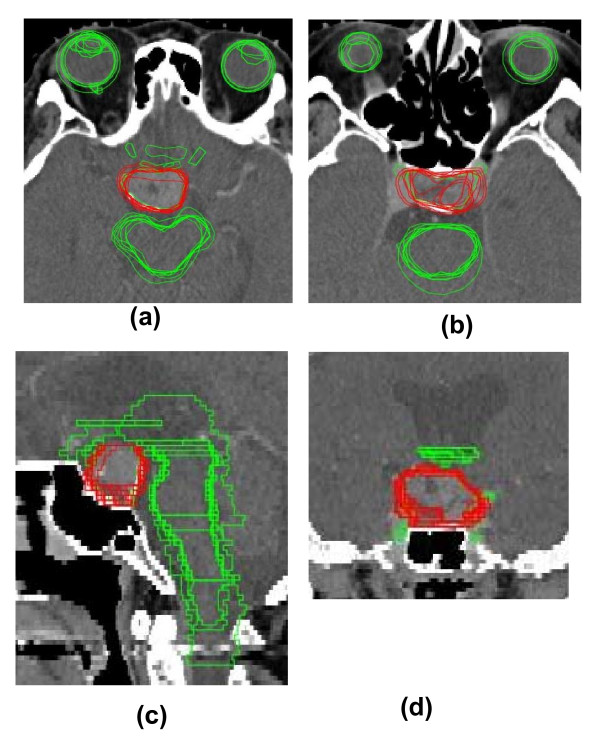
Contours superimposed on the default CT image (Pituitary adenoma). (a) (b) axial section (c) sagittal section (d) coronal section.
